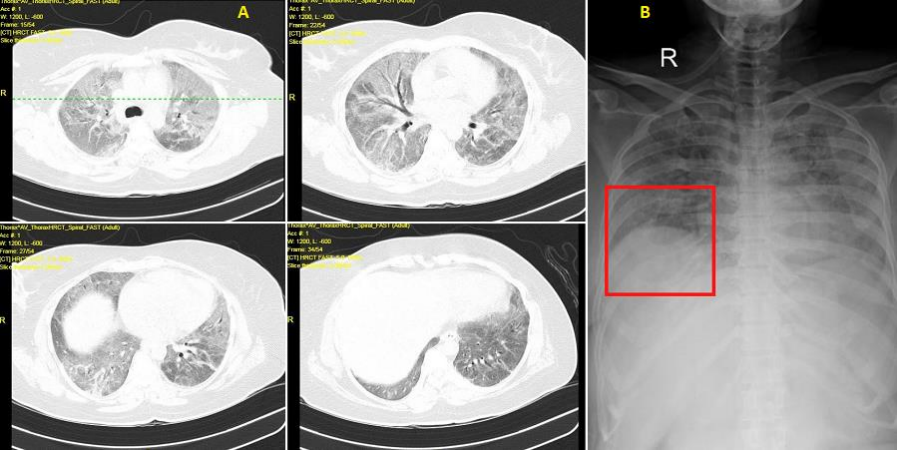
A) multiple ill-defined patchy ground-glass opacities with consolidation, septal thickening, and fibrotic changes in bilateral lung fields suggestive of COVID-19 changes with atypical viral pneumonia with CT severity score 25/25; B) bilateral opacities seen in bilateral lobes and right hemi diaphragm is pulled up
